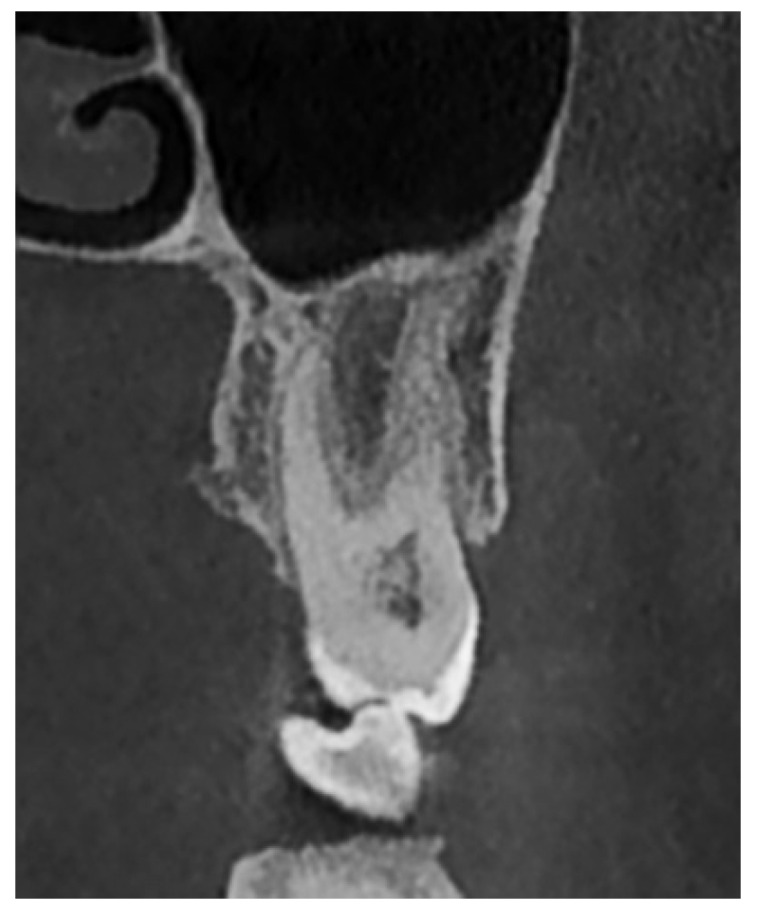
Cross-sectional CBCT reformat along the long axis of tooth 27. Close-type relationship between tooth 27 and the maxillary sinus.
